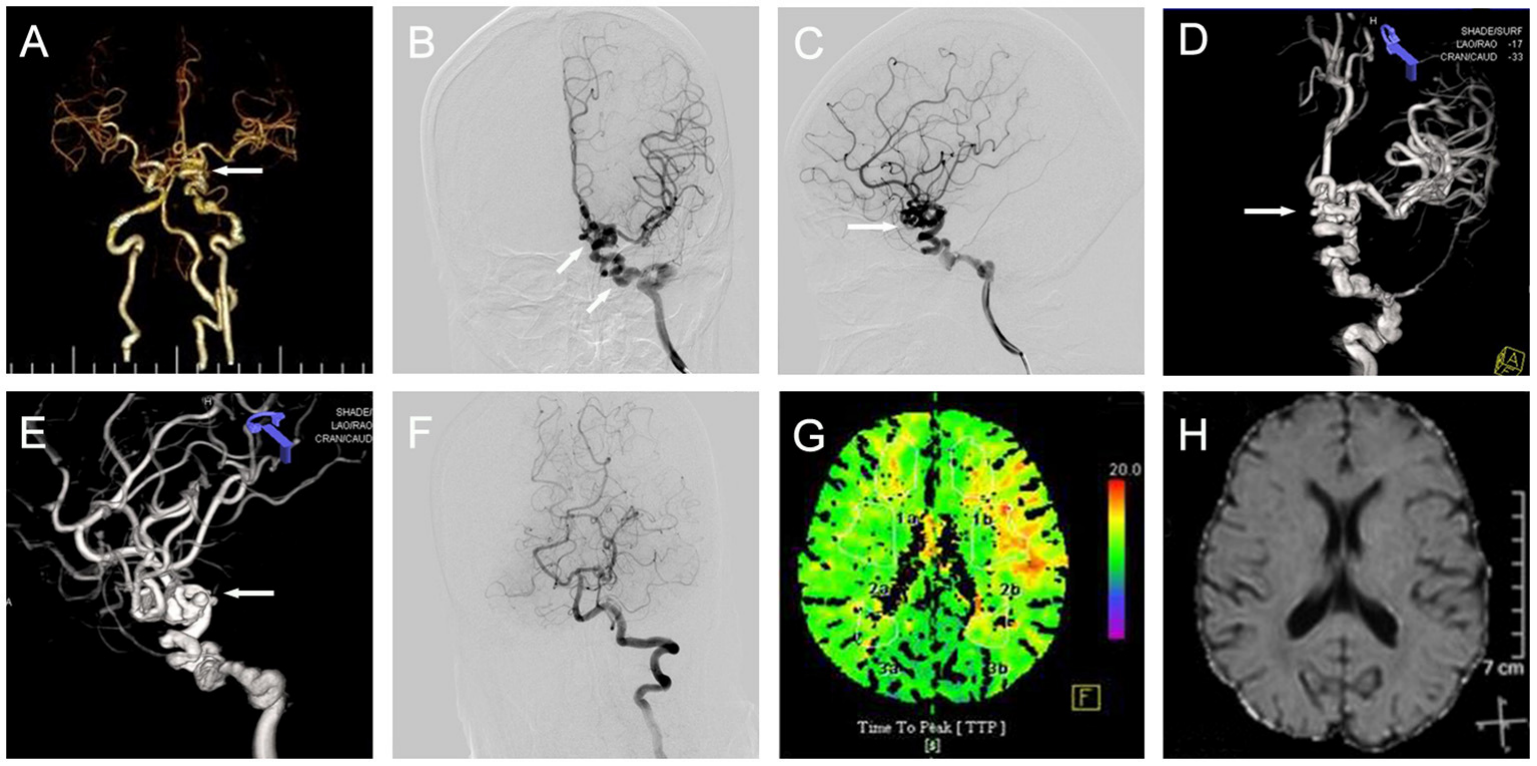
Case 1. Three-dimensional reconstruction of CT angiogram showing a dilated, overlapping, and tortuous [(A), arrow] left ICA, [(B), arrow] anteroposterior, [(C), arrow] and lateral DSA views; and three-dimensional anteroposterior [(D), arrow], and lateral [(E), arrow] DSA views. (F) Anteroposterior view of left vertebral artery injection angiograms. (G) Axial CTP showing increased TTP in the left hemisphere. (H) Axial DWI MRI showing the absence of ischemic stroke. ICA, internal carotid artery; CT, computed tomography; DSA, digital subtraction angiography; DWI, diffusion-weighted imaging; MRI, magnetic resonance imaging; TTP, time to peak; CTP, computed tomography perfusion.
